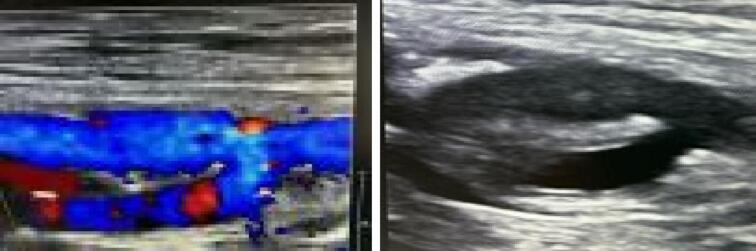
The duplex sonography control was performed at three and six months. The femoral axis remained consistently patent without signs of recurrent occlusion or stenosis throughout.
